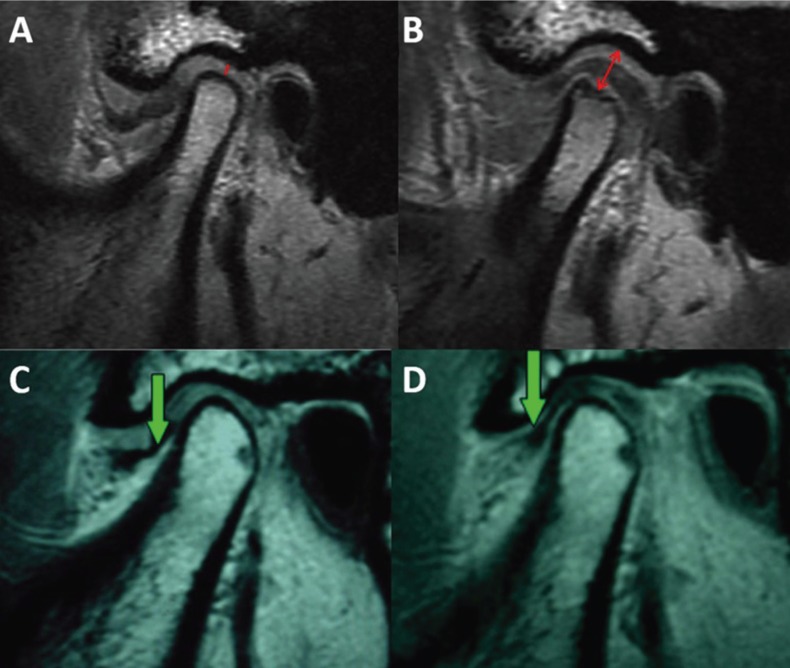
A and B) Magnetic Nuclear Resonance Imaging (MRI) taken from the article Hu *et al.*, (2017)(11)- demonstrating arthroscopic surgery with disc plication. A) Preoperative with decreased joint space and anteriorly displaced disc. B) Postoperative with repositioning of the disc and increased joint space with decompression (red line), where there will necessarily be occlusal alteration. It is also observed that the joint cartilage is reabsorbed by probable progression of arthropathy or disc compression on the cartilage. C and D) Images taken from Zhang *et al.*, (2010)(15]. C) MRI in the preoperative arthroscopic surgery evidencing anterior disc displacement (green arrow) and reduction of joint space. D) Postoperative of arthroscopic disc repositioning (green arrow) and increased joint space.
